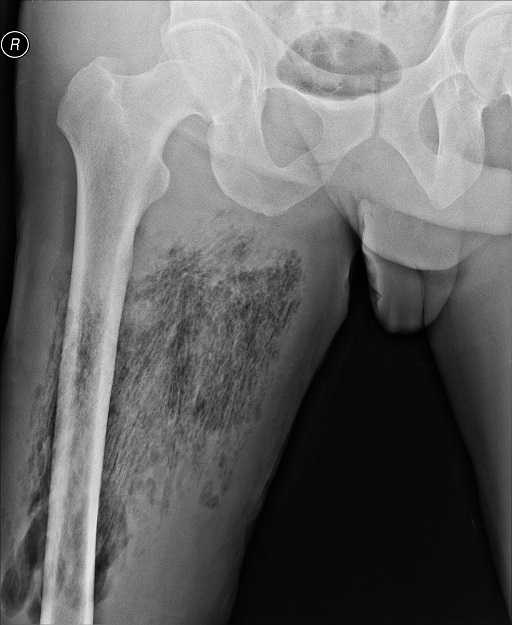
Plain x-ray of the thigh demonstrating a feathering pattern indicative of the presence of gas in the soft tissue
